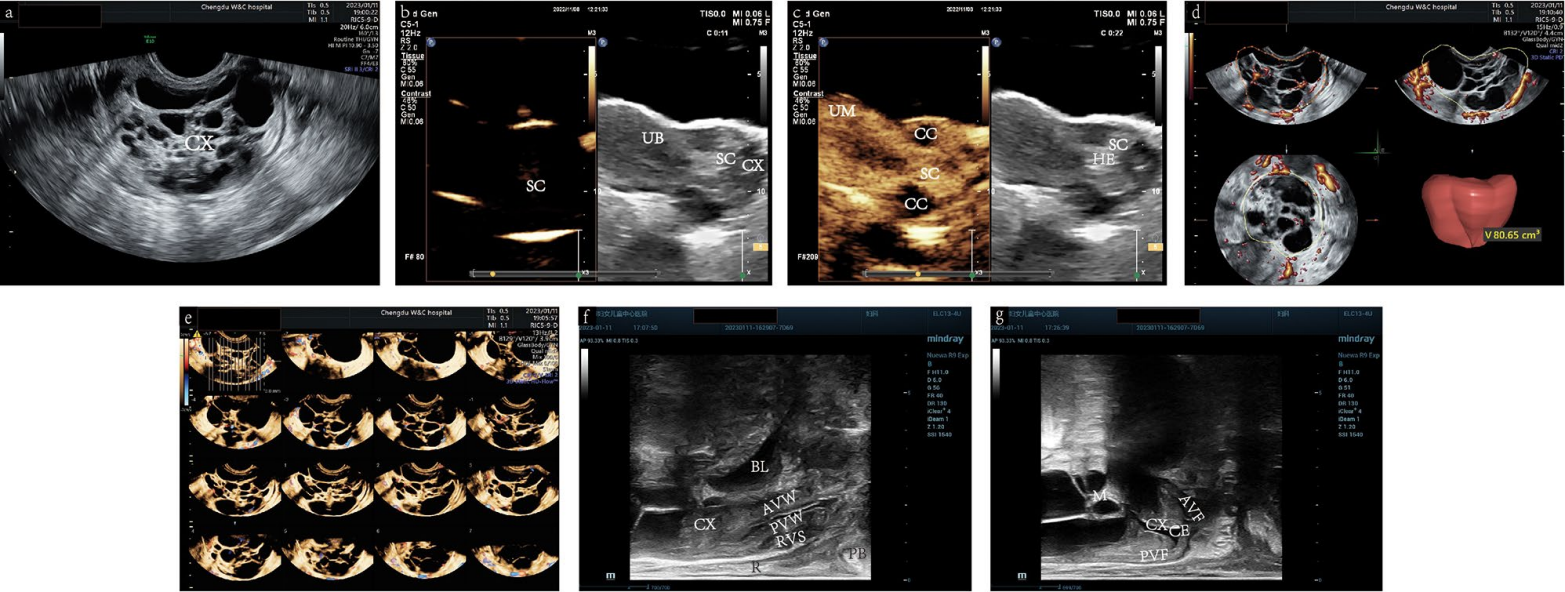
Multimodal ultrasonography of cervical lesions. (a) Transrectal gray-scale US (GE voluson E10 RIC5-9-D, General Electric Company, Boston, Massachusetts, the United States) showing an enlarged cervix with multiple cystic lesions of various sizes and a few solid components. The solid components are hyperechoic. The cystic lesions are irregular, honeycomb-shaped, and of various sizes and were encompassed by relatively larger cysts. (b) Transabdominal CEUS ((PHILIPS EPIQ7, Philips Healthcare, Seattle, Washington, the United States) showing perfusion of the solid components of the cervical lesions before the myometrium. (c) Transabdominal CEUS image showing an unevenly enhanced uterine myometrium, unenhanced cystic echoes, and equally enhanced solid components when peaked. (d) Measurement of the mass volume using VOCAL software. (e) Three-dimensional TUI (General Electric Company, Boston, Massachusetts, the United States) of blood flow in the cervical lesion indicates that its blood supply is located in the intercyst septate and solid components. (f) Biplane transrectal US sagittal view using Biplane Endocavity convex-linear array transducer (ELC13-4U, Mindray, Shenzhen, Guangdong, China) revealing the normal structure of the vagina without penetration of the cancerous lesion. (g) Biplane transrectal US sagittal view showing intact and smooth rims of the anterior and posterior vaginal fornices without thickening or penetration of the lesion. *Abbreviation* AVF, anterior vaginal fornix; AVW, anterior vaginal wall; AVW, anterior vaginal wall; BL, bladder; CC, cystic component; CE, cervical effusion; CEUS, contrast-enhanced ultrasonography; CX, cervix; HE, hyperecho; PB, perineal body; PVF, posterior vaginal fornix; PVW, posterior vaginal wall; PVW, posterior vaginal wall; R, rectum; RVS, rectovaginal septum; SC, solid component; TUI, tomographic ultrasound imaging; UM, uterine myometrium; US, ultrasound
